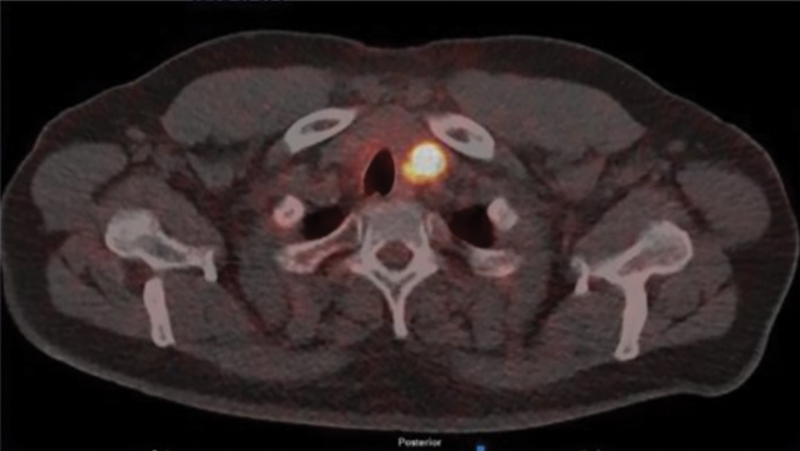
Axial prostate-specific membrane antigen (PSMA)-positron emission tomography (PET)-computed tomography (CT) images showing high-grade uptake in the left thyroid nodule.
